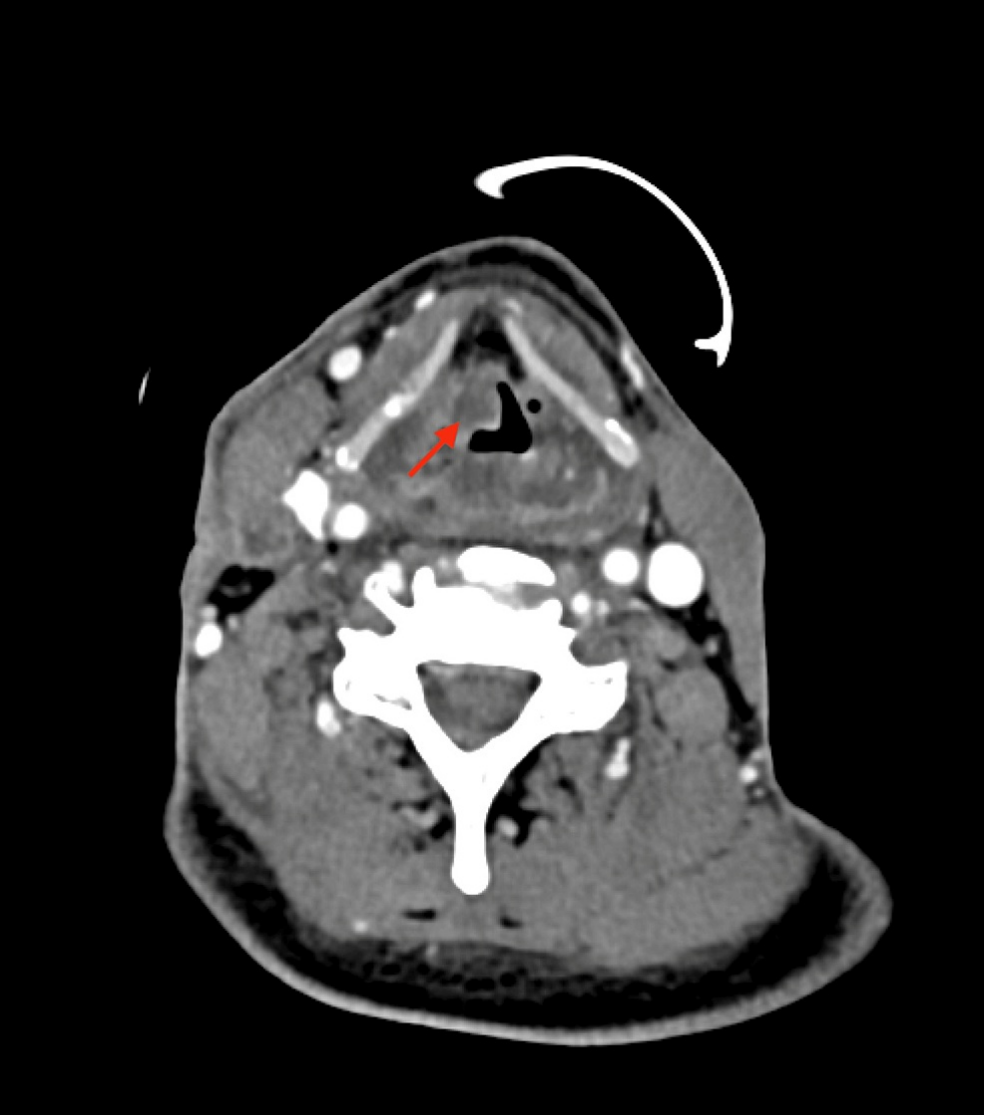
Neck (soft tissue) CT with IV contrast demonstrating the transglottic laryngeal mass (axial view)
